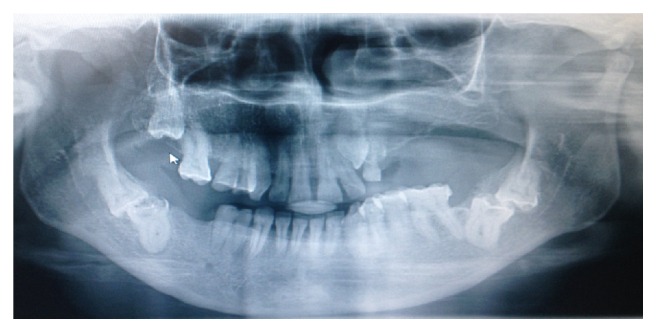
Orthopantomography showing the presence of kissing molars in each side of the mandible.
